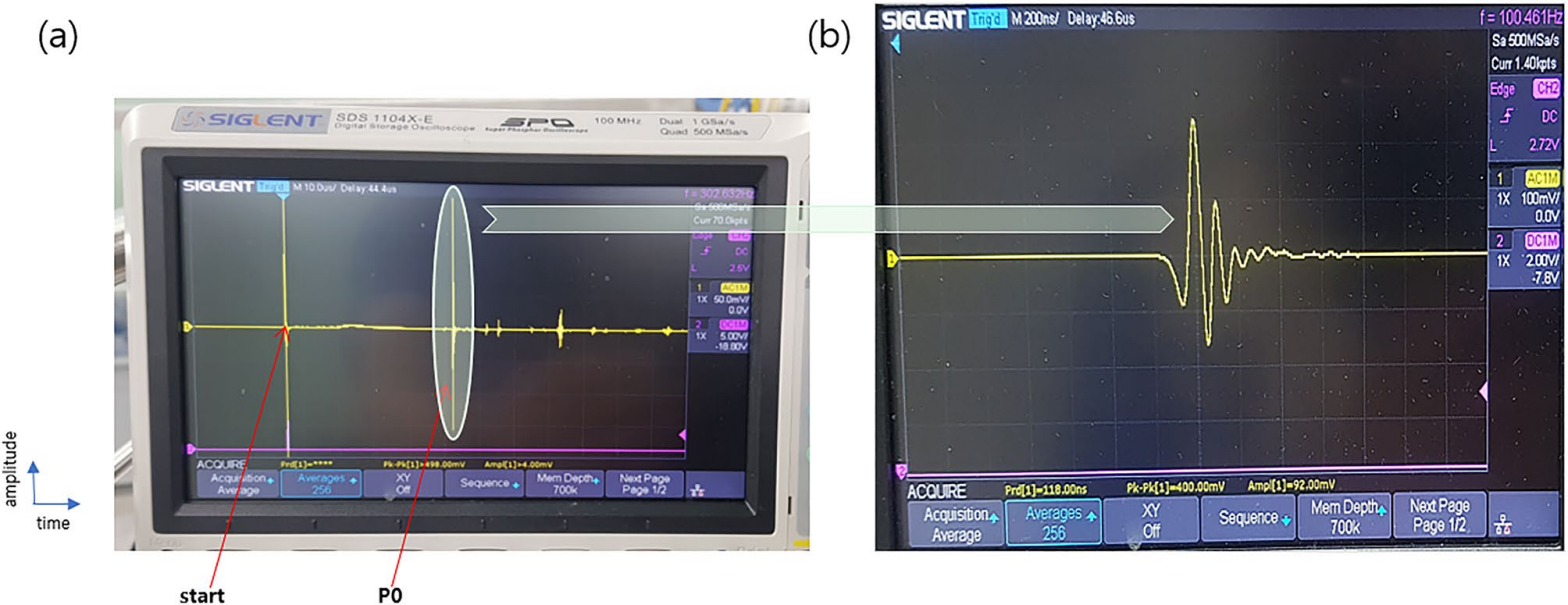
Ultrasonic waveforms at the DSO. (A) Full waveform showing multiple ultrasonic pulses with different paths through the ultrasonic chamber (B) Magnified ultrasonic pulse waveform with the lowest travel time, first arrived pulse wave (P0).
